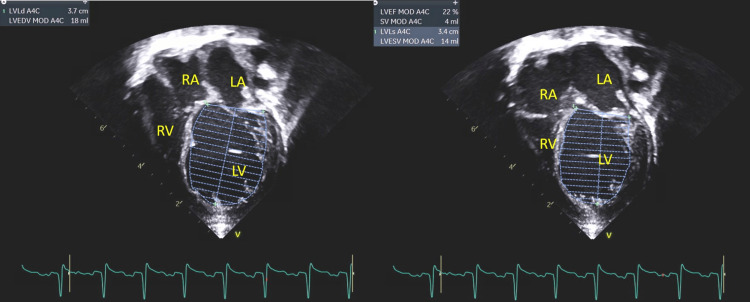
Apical 4 Chamber view showing severely dilated LV with LVEF 22% done at three weeks of age. LVEF: Left ventricle ejection fraction
